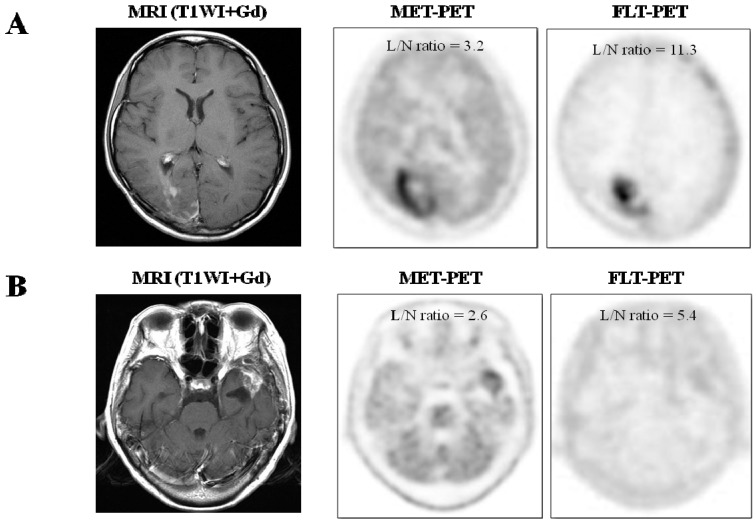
(A) Imaging of a 22 year-old female (case 9) with glioblastoma, previously treated with tumor resection followed by conventional radiotherapy and temozolomide. T1-weighted contrast enhanced MR image showing irregular enhancement in the right occipital lobe. MET-PET and FLT-PET show intense uptake of tracer in the lesion. Recurrent glioblastoma was pathologically confirmed by surgery; (B) Imaging of 61 year-old female (case 21) with glioblastoma, previously treated with tumor resection followed by conventional radiotherapy and temozolomide. T1-weighted contrast enhanced MR image showing enhanced mass in the left temporal lobe. MET-PET shows mild uptake of tracer and FLT-PET shows faint uptake of tracer in the lesion. Necrosis and gliosis dominant tissue was pathologically confirmed by surgery.
